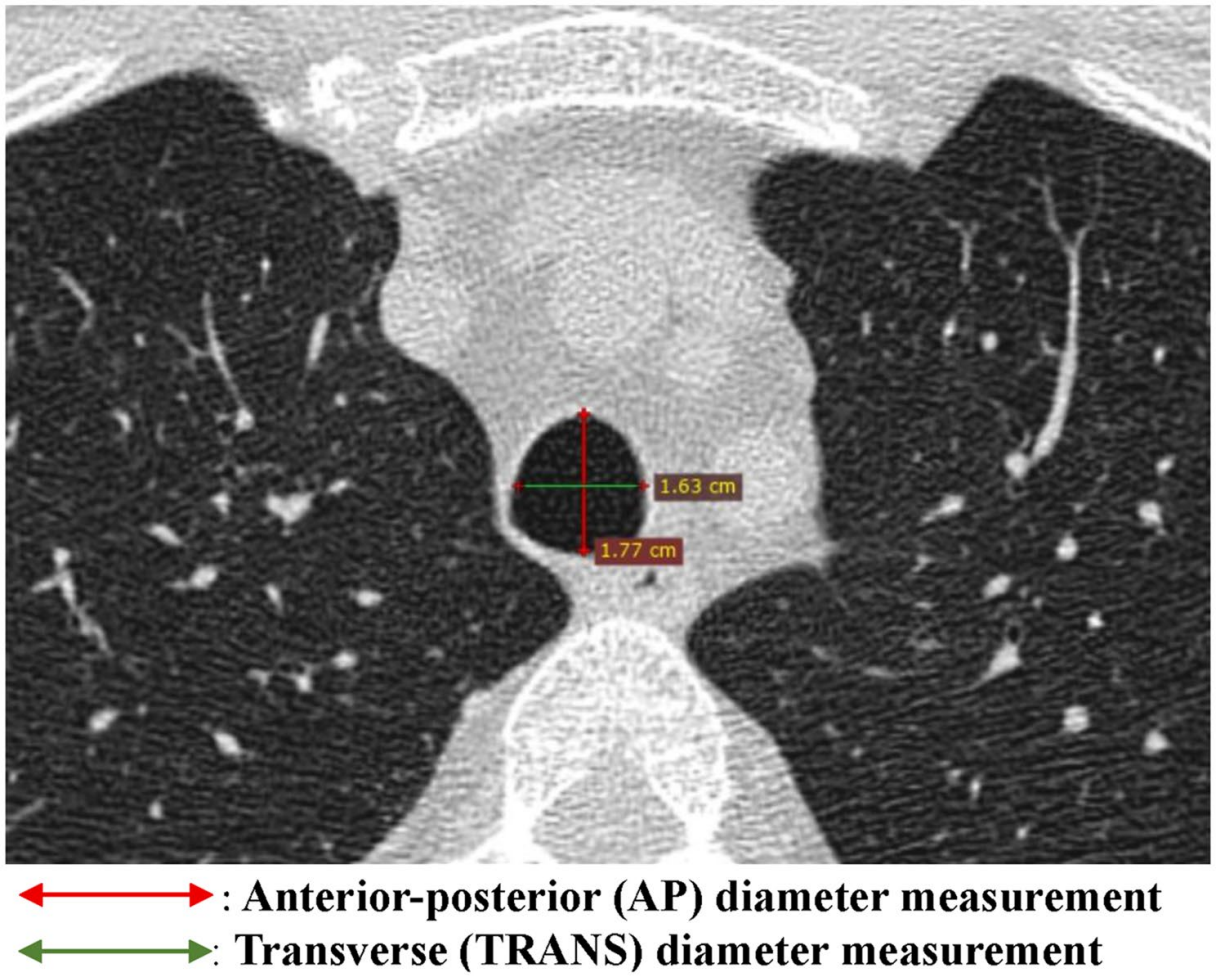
Axial chest CT reconstruction (lung window) demonstrating tracheal diameter measurements
